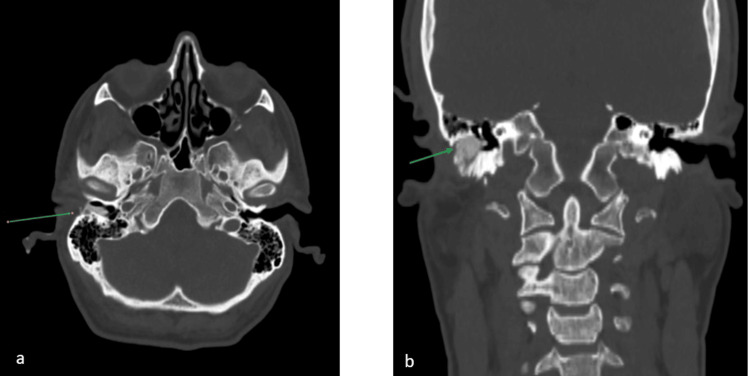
CT fistulography The green arrows reveal the fistula and its relation to the external auditory canal; (a) axial view, (b) coronal view.
